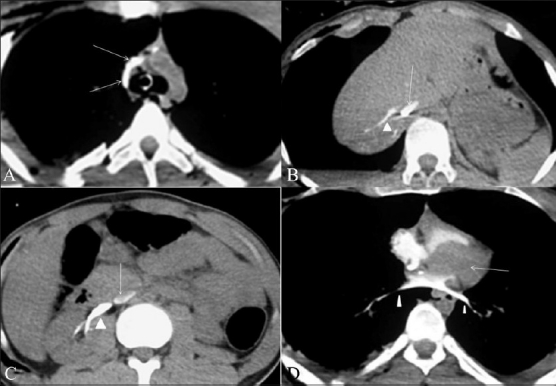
Cardiac arrest in a 25-year-old male with polytrauma. Axial contrast-enhanced CT scans show intense opacification of the SVC and azygos vein (arrows in A). Contrast is seen pooling in the dependent part of the IVC (arrows in B and C), with opacification of the hepatic veins (arrowheads in b) and the right renal vein (arrowhead in C). There is no contrast in the left heart chamber (arrow in D). Contrast opacification of the pulmonary veins is seen (arrowheads in D). Note that there is no parenchymal enhancement of the liver or kidney and no contrast is seen in the aorta (B,C)
